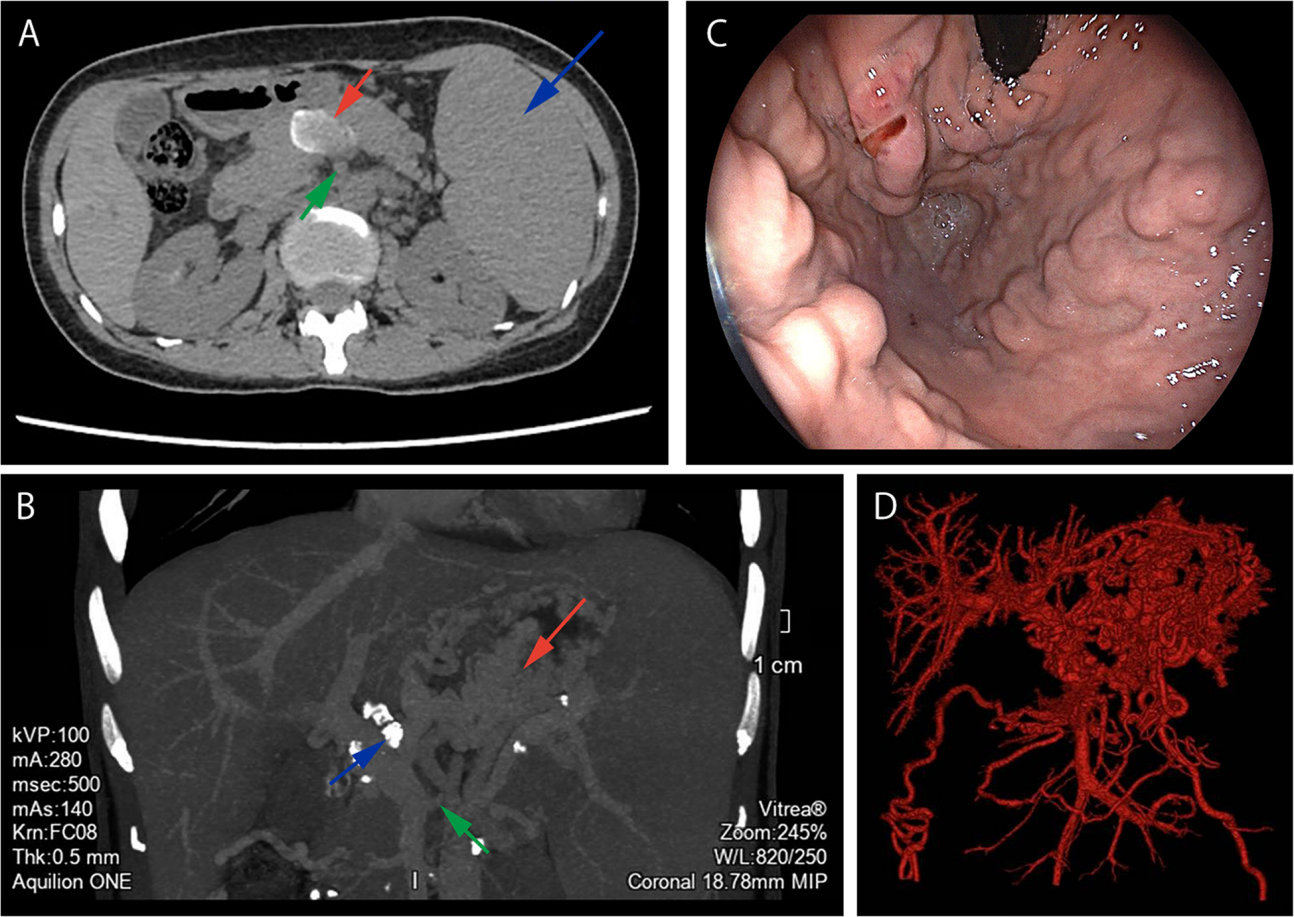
Computed tomography and gastroscopy images. a Non-contrast computed tomography imaging on a horizontal plane. There is a low-density and heterogeneous mass in the pancreas body with an irregular margin (red arrow). The spleen is enlarged (blue arrow), and there are dense nodular shadows around the pancreas (green arrow). b Computed tomography angiography image in the portal venous phase obtained in a coronal plane. Varicose gastric veins (red arrow) and swollen calcified lymph nodes (blue arrow) are evident. The initial section of the splenic vein becomes narrow (green arrow). c Gastroscopy image depicting a varicose vein in the gastric fundus. d Portal-phase three-dimensional vascular reconstruction image depicting varicose gastric veins and normal esophageal portal veins
